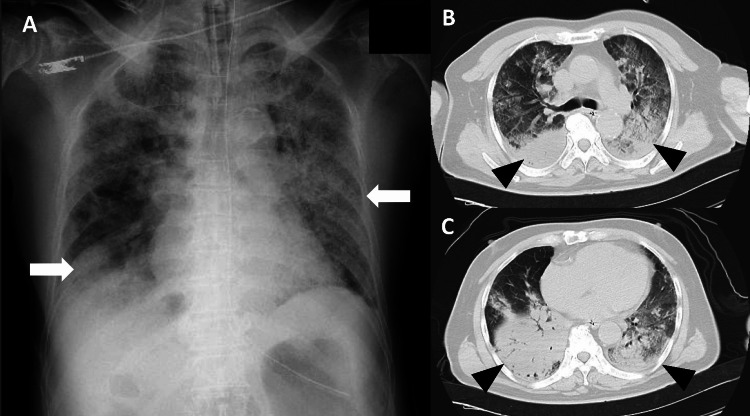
Images of chest x-ray and computed tomography (CT) Chest radiograph showing extensive consolidation and ground-glass opacity in both lungs (A, white arrows), and the axial view of the chest CT scan showing infiltrates in the peripheral areas of the bilateral lower lobes in addition to similar findings on radiography (B, C, black arrowheads).
